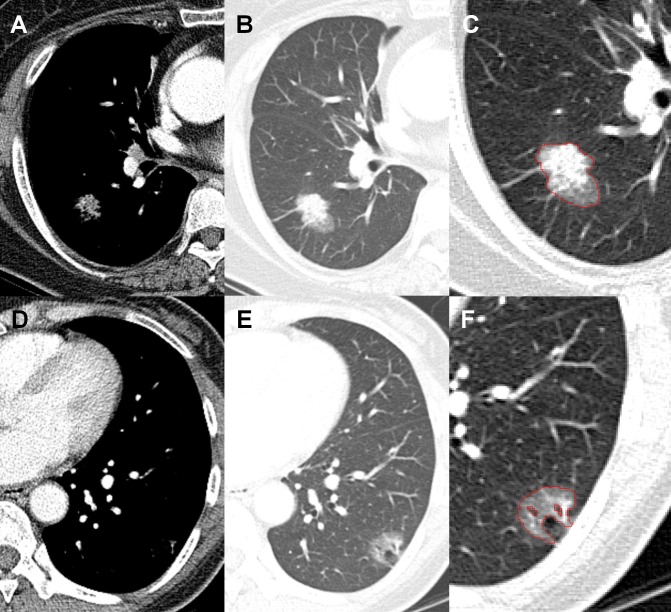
Part-solid adenocarcinomas with or without recurrence. (A) Mediastinal setting and (B) lung setting of CT images show a 3.2-cm part-solid adenocarcinoma in the right lower lobe (T2aN0M0, stage Ib). The lesion shows -315.27 HU (Hounsfield unit) and the area of lesion was 443.95 mm^2^. (C) The region of interest (ROI) for the tumor drawn in the ImageJ was noted. Lung metastasis was developed two years after the surgery. (D, E) A 2.1-cm part-solid nodule in the left lower lobe. The tumor area was 247.65 mm^2^ and the attenuation was -445.93 HU. (F) Air-bronchogram within the lesion was excluded for the measured ROI of the lesion. There was no recurrence in this patient for recent three years.
